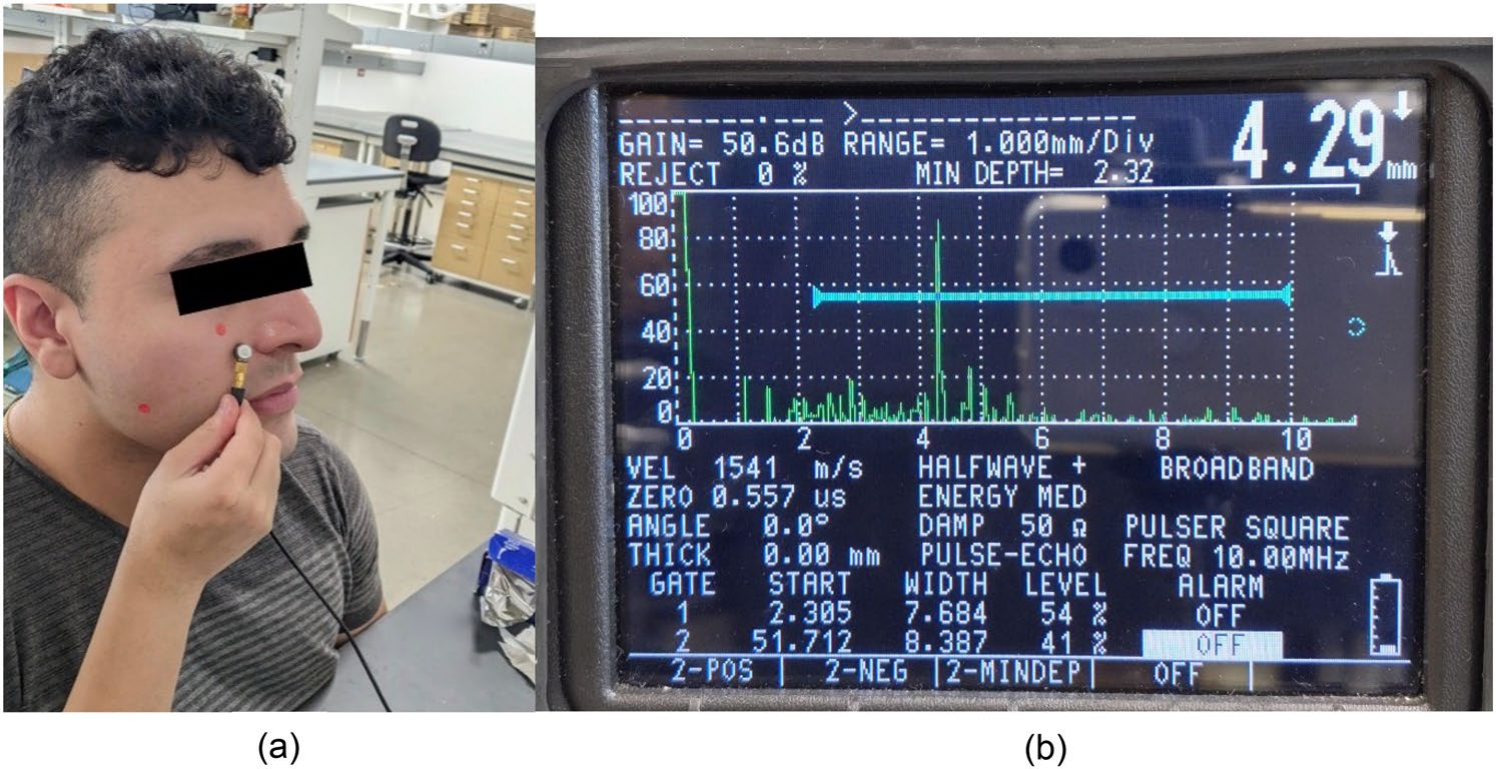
Ultrasound testing on a subject (a) and example result on ultrasound display (b)
